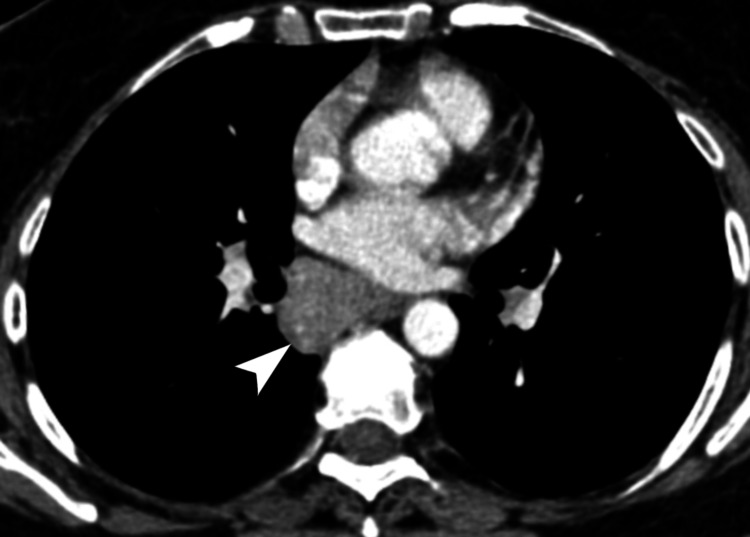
Subcarinal nodal mass with esophageal and airway encasement. Axial CT of the chest, in the soft tissue window, demonstrates a homogeneously enhancing nodal mass in the subcarinal region (white arrowhead), measuring 4.7 × 2.5 × 5.8 cm (transverse × AP × craniocaudal), partially encasing the thoracic esophagus and both the right and left main bronchi. CT: computed tomography
